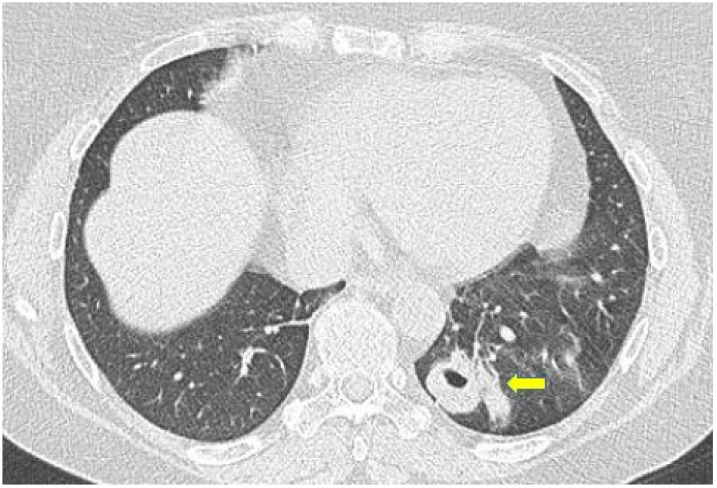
Axial high resolution CT scan in a female patient with Granulomatosis with polyangiitis shows nodule with cavitation presenting thick walls and irregular margins (yellow arrow).
